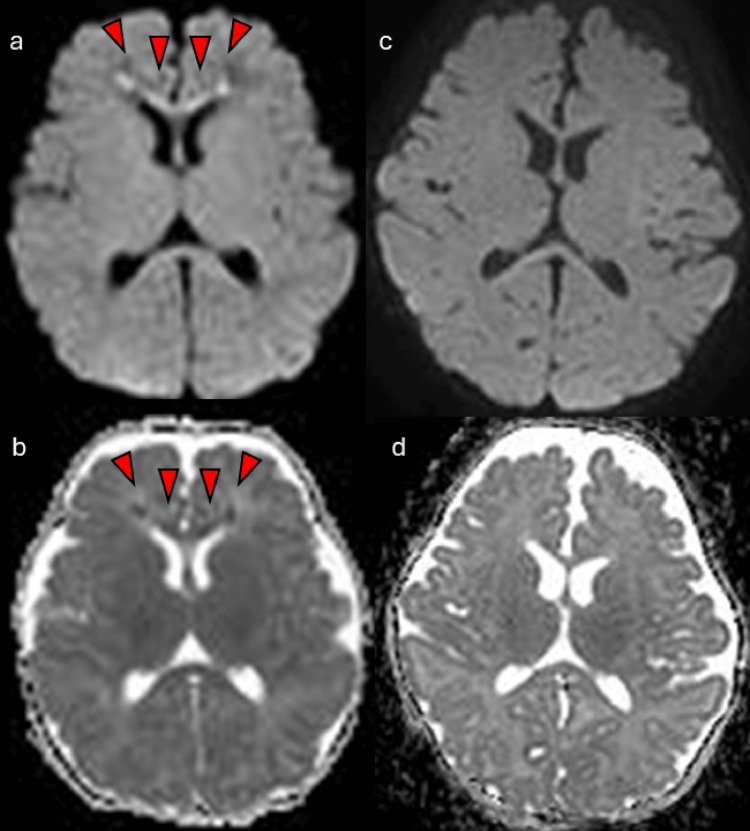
Serial brain MRI findings. Upper row: Diffusion-weighted imaging (DWI).
